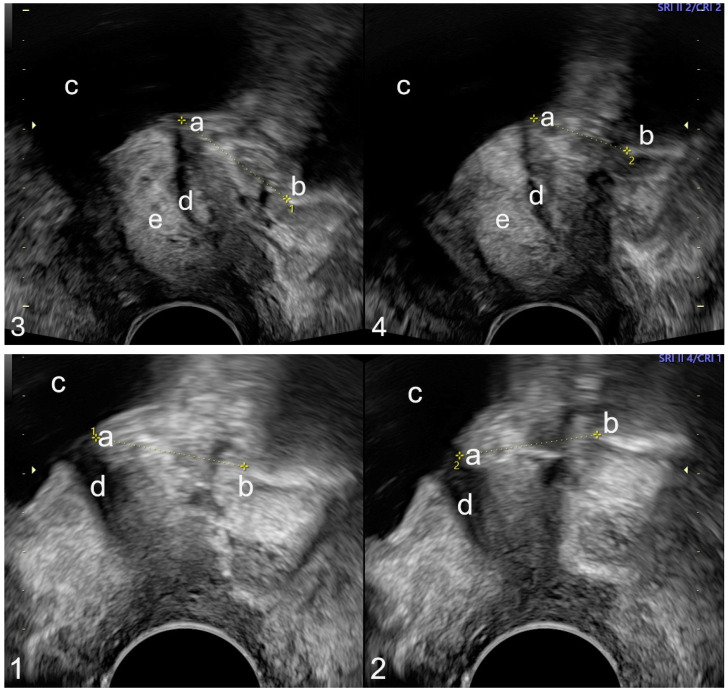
Pelvic floor sonography was performed using a 2D vaginal probe with a frequency range of 3.5–10 MHz (GE^®^, model Voluson E8, Wien, Austria), targeting the internal urethral orifice (a), symphysis pubis edge (b), bladder (c), and urethra (d). Imaging was conducted preoperatively (1,2) and postoperatively (3,4) to assess urethral mobility via linear dorsocaudal movement (LDM, yellow dashed line), utilizing the hyperechoic Bulkamid^®^ (e) three days after Bulkamid^®^ injection. Specifically: (1) preoperative at rest (LDM 19 mm), (2) preoperative under stress (LDM 18 mm), (3) postoperative at rest (LDM 20 mm), and (4) postoperative under stress (LDM 16 mm).
